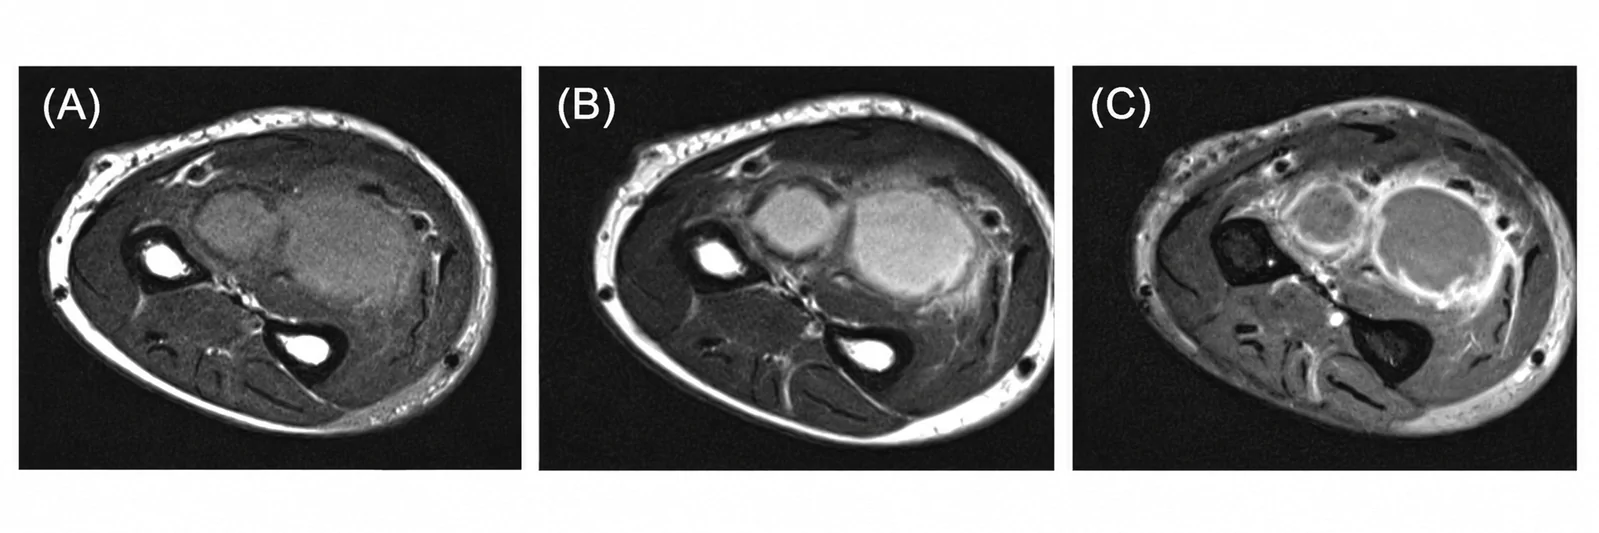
MRI Findings of the Right Forearm Mass Right forearm MRI at presentation. (A) Axial T1-weighted image shows a mildly hyperintense intramuscular lesion (arrows). (B) Axial T2-weighted image shows marked lesion hyperintensity (arrows) with extensive surrounding edema. (C) Axial fat-suppressed contrast-enhanced image shows peripheral rim enhancement of the lesion (arrows) and enhancement in the surrounding edematous tissue.
